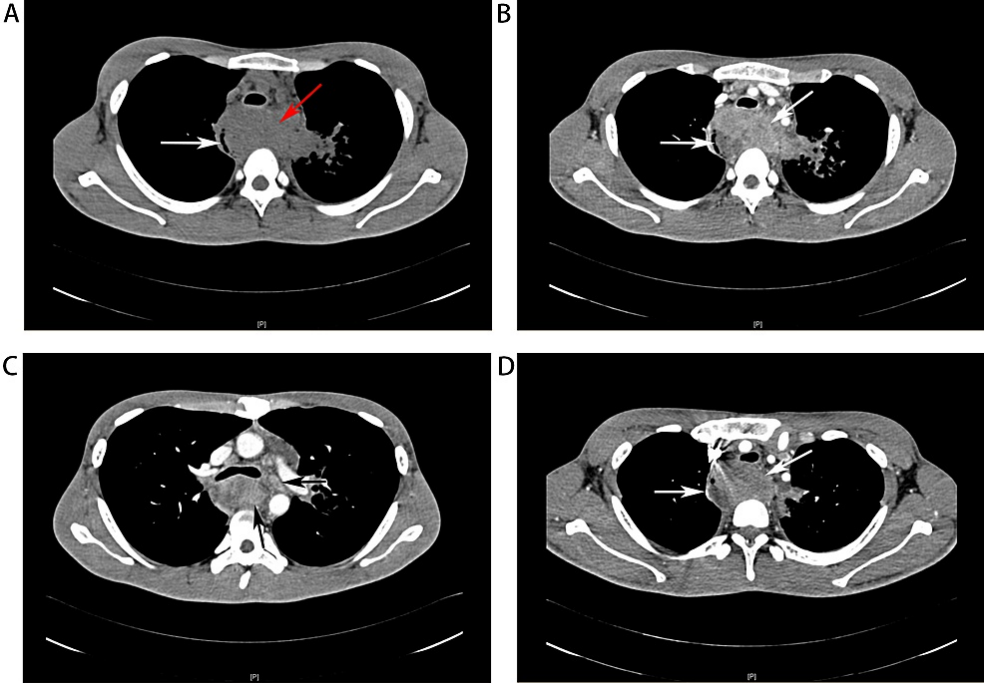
Imaging characteristics of primary esophageal lymphoma (A) CT revealing that the lesion was a low-density mass with obvious thickening of esophageal wall (red arrow) and stenosis of esophageal lumen (white arrow). (B) Contrast-enhanced CT revealing a mild heterogeneous enhanced lesion during the venous phase (white arrow). (C) Contrast-enhanced CT showing a mediastinal lymph node enlargement (black arrow), fusion with esophageal lesions, unclear boundary during the venous phase. (D) Contrast-enhanced CT showing a heterogeneous enhanced lesion during the arterial phase (white arrow).
